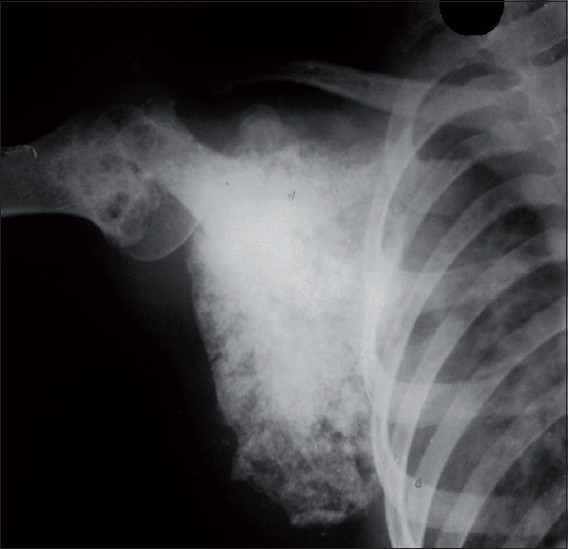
Radiograph of right shoulder showing extensive sclerosis of the lesion after chemoradiation
